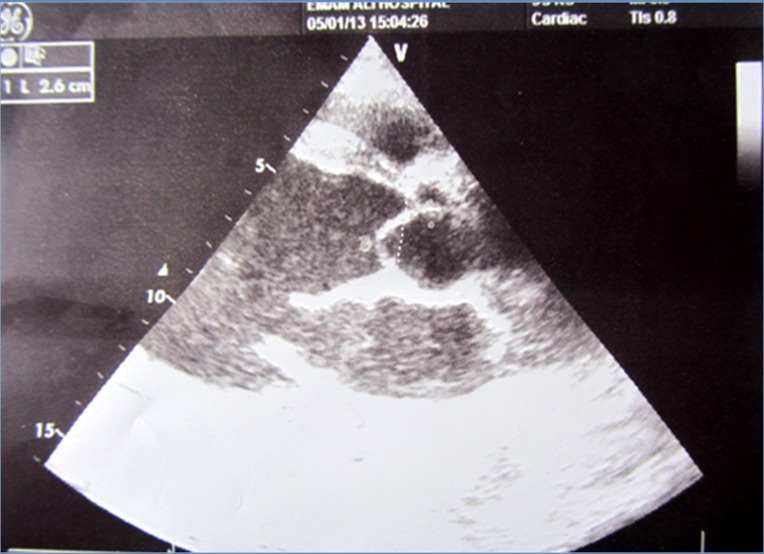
Transesophageal echocardiography revealing a flail aortic valve.
